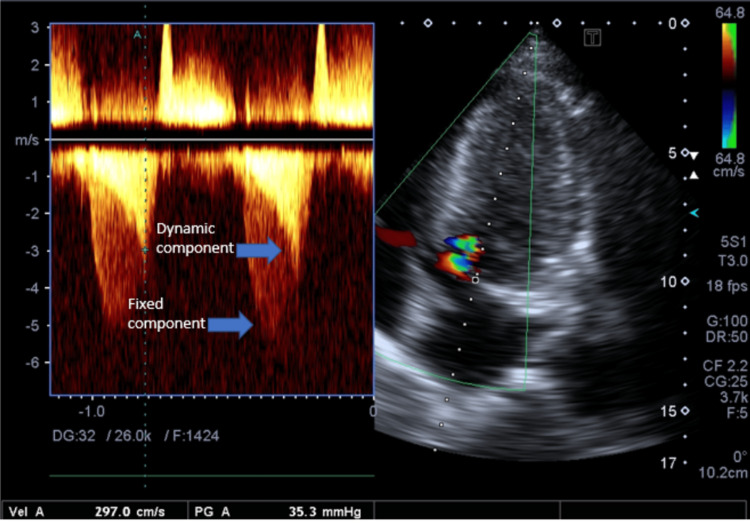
AP5CH view with color Doppler and spectral Doppler image There is double envelope of LVOT flow showing high flow velocities and gradients of dynamic and fixed components. Dynamic peak velocity 2.97m/sec and peak gradient 35mmHg. AP5CH: apical five chamber; LVOT=left ventricular outflow tract
